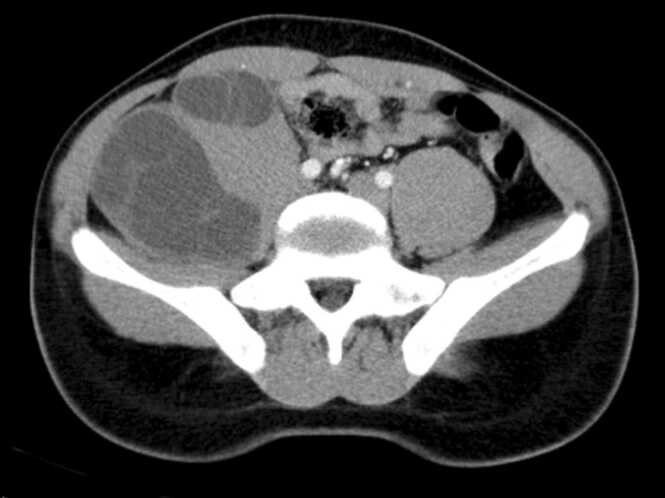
Axial view of abdominal CT scan showing the two psoas muscle hydatid cyst.
